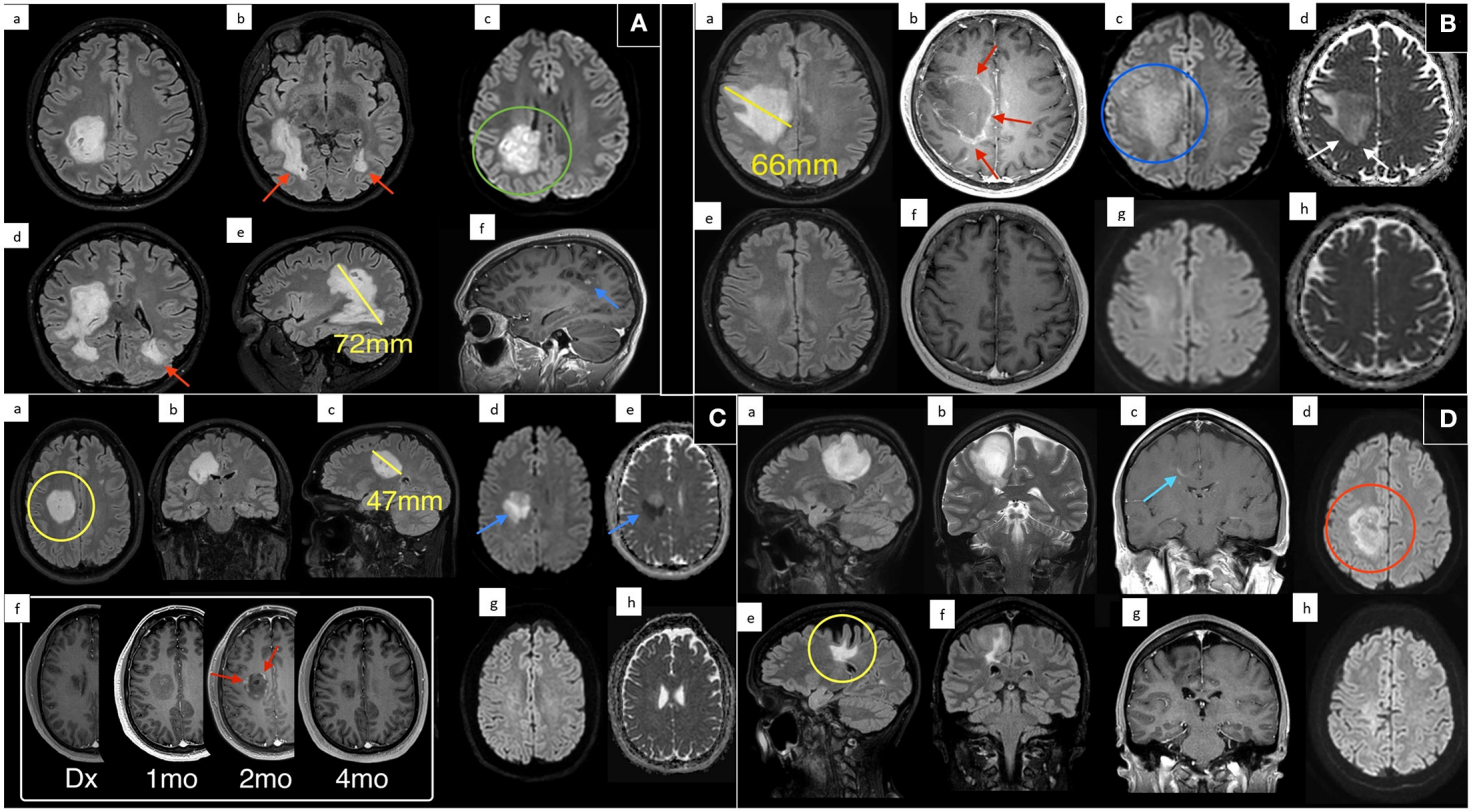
Magnetic resonance imaging of each patient at presentation and various treatment time points. (A) Case 1: MRI following presentation demonstrates a giant hyperintense confluent right sided periventricular lesion on axial T2 FLAIR (a/b), with coronal oblique T2 FLAIR reconstruction (d), and sagittal oblique (e) reconstruction. Maximum dimension of the dominant lesion measuring up to 72 mm on sagittal imaging. Evidence of internal diffusion hyperintensity (green circle) on axial DWI (c) and internal punctate foci of enhancement (blue arrow) on sagittal T1 post contrast (f). There was evidence of further periventricular T2 FLAIR hyperintense lesion adjacent to the contralateral left occipital horn (red arrows). (B) Case 2: MRI at presentation (a–d) and 4 months follow up (e–h). Scan at diagnosis demonstrates a large solitary T2 FLAIR (a) hyperintense lesion centered on the right frontal white matter with no significant mass effect. This lesion measured up to 66 mm with peripheral enhancement (red arrows) on post contrast T1 imaging (b) with patchy internal diffusion restriction (blue circle) on DWI (c), and subtle peripheral restricting peripheral margin (white arrow) on ADC (d). Near complete resolution on follow up imaging with minimal residual T2 FLAIR hyperintensity (e). (C) Case 3: MRI at presentation (a–e), multiple follow up times points post contrast T1 imaging (f), and 4 month follow up DWI (g) and ADC (h). On multiplanar T2 FLAIR (a–c) there is a large periventricular lesion (yellow circle) measuring up to 47 mm with marked associated diffusion restriction (blue arrows) on DWI (d) and ADC (e). No enhancement was demonstrated on scan at diagnosis (c) however the patient progressively developed classic interrupted peripheral enhancement (red arrows). On delayed 4 month follow up scan enhancement and diffusion restriction had resolved (g/h). (D) Case 4: MRI at presentation with sagittal T2 FLAIR (a), coronal T2 (b), coronal T1 post contrast (c), and DWI (d) demonstrates a large T2/FLAIR hyperintensity extending from the periventricular margin to the cortex of the right posterior frontal lobe. There was wispy associated linear enhancement (blue arrow) at diagnosis on post contrast T1 imaging (c) and regions of internal and peripheral diffusion restriction (red circle) on DWI (d). Six year follow up scan (e–h) shows marked reduction in FLAIR signal, some associated cortical atrophy and volume loss (yellow circle) and resolution of previous enhancement and diffusion restriction.
